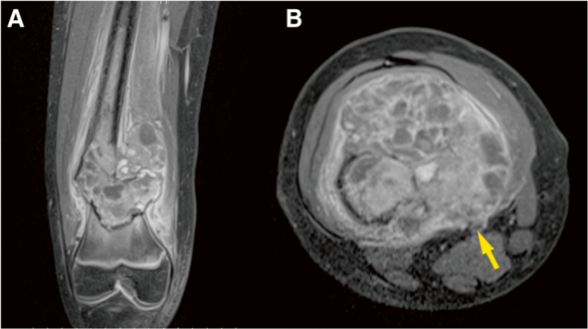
Preoperative contrast-enhanced magnetic resonance image. a Coronal view. b Axial view. Superficial femoral vessels (*arrow*)
